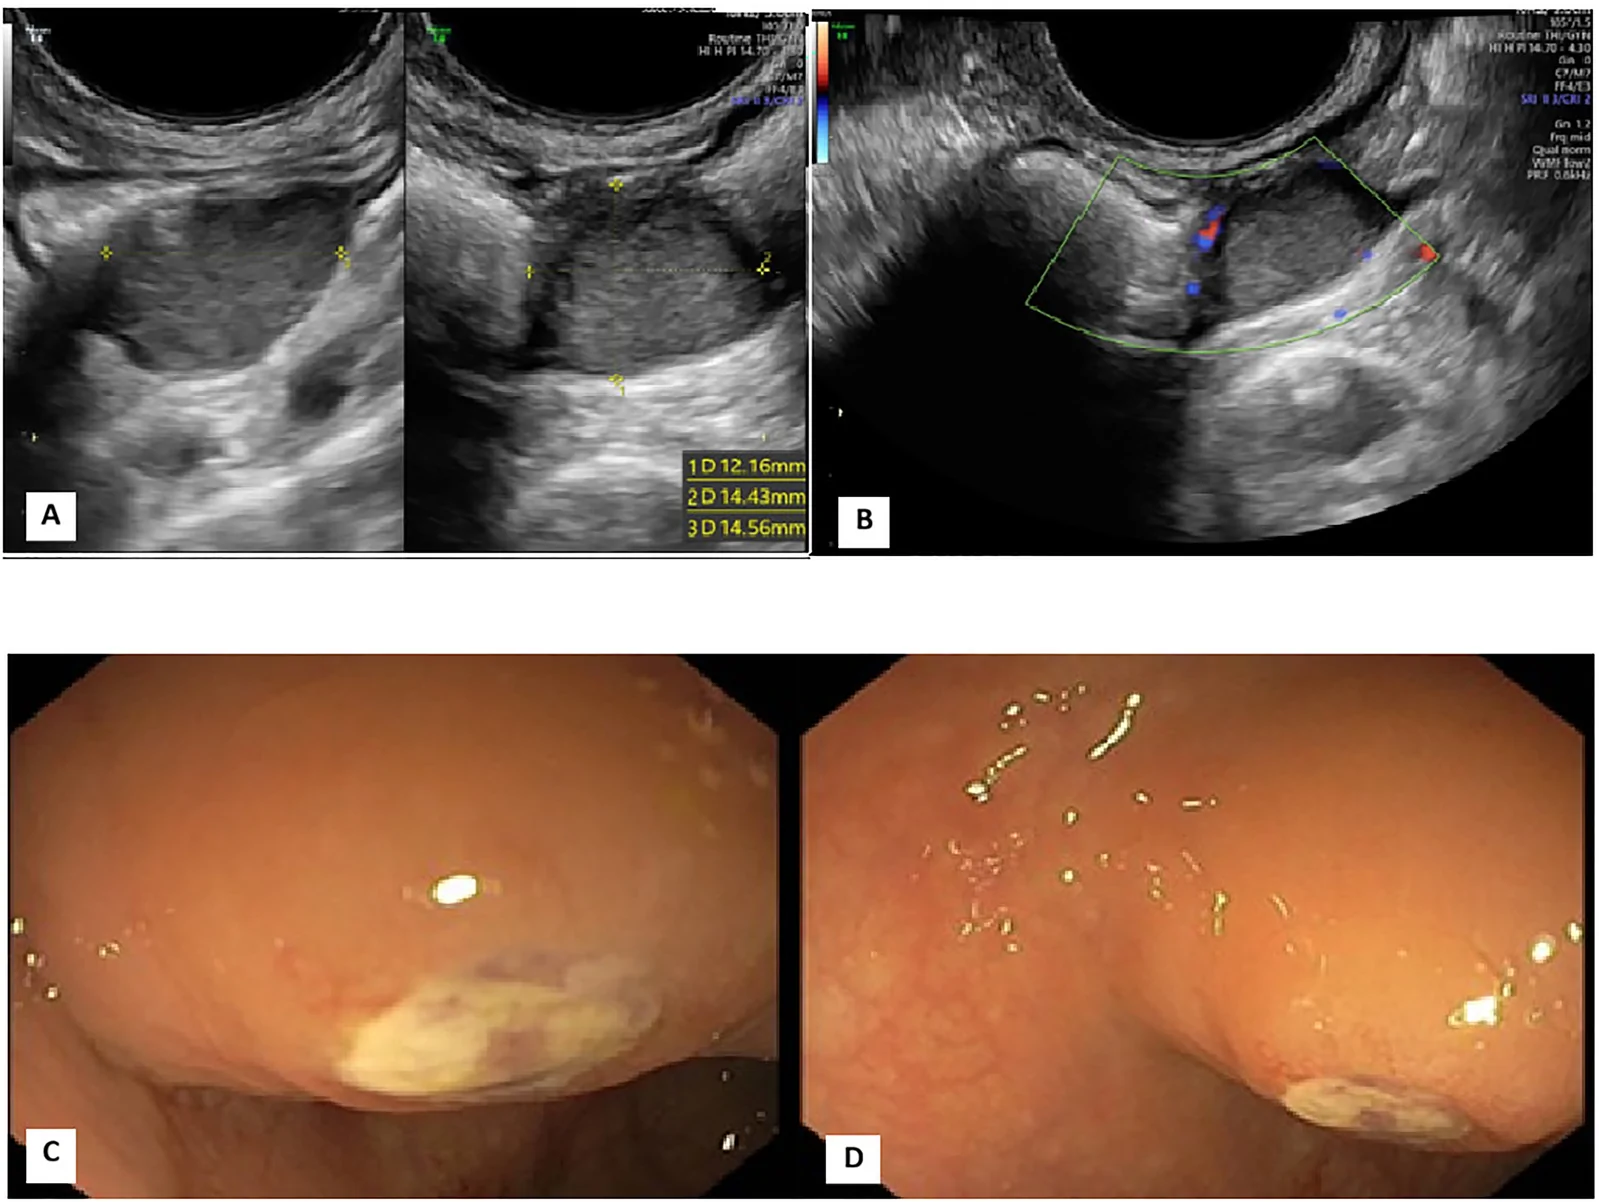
(A, B) Transvaginal ultrasound images of the lesion showing a solid mass arising from the lateral wall of the sigmoid colon, showing internal vascularization (CS2); (C, D) Endoscopic vision of an ulcerated sigmoid lesion.
